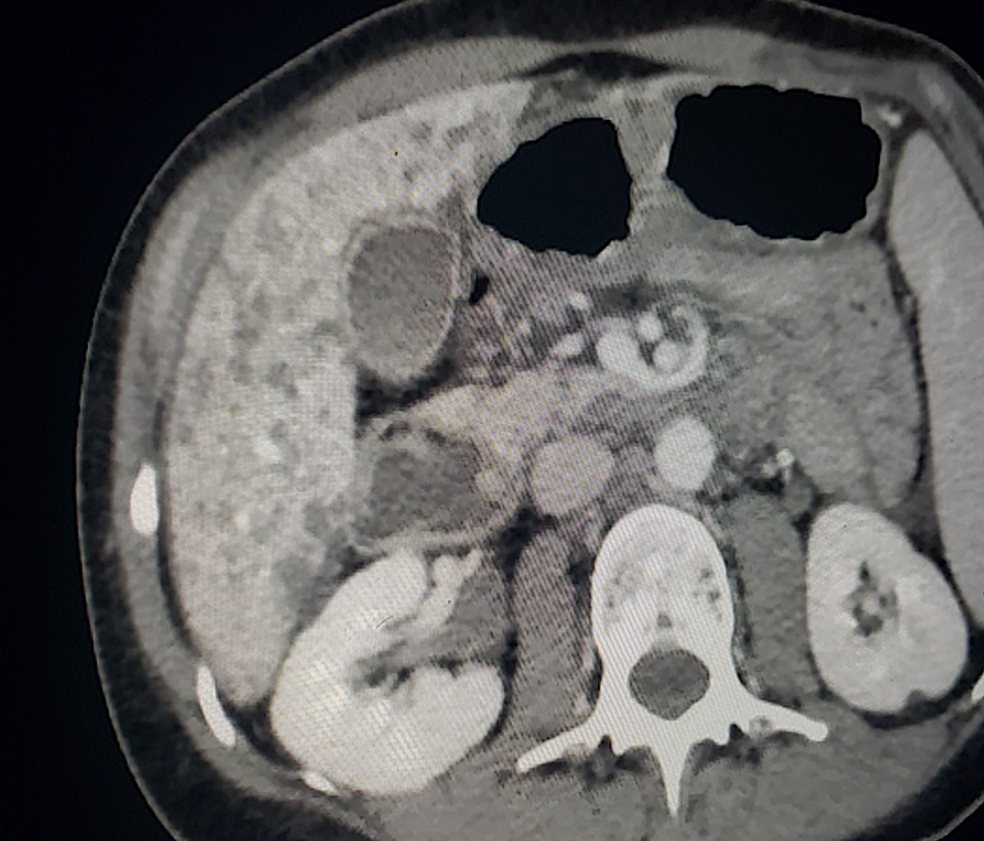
Computerized tomography of abdomen and pelvis with IV contrast. There were innumerable small hypodensities throughout the liver, new from a prior PET CT imaging five months prior. These were concerning for microabscesses from bacterial or fungal infection. Nonspecific adjacent inflammatory changes were also apparent.
